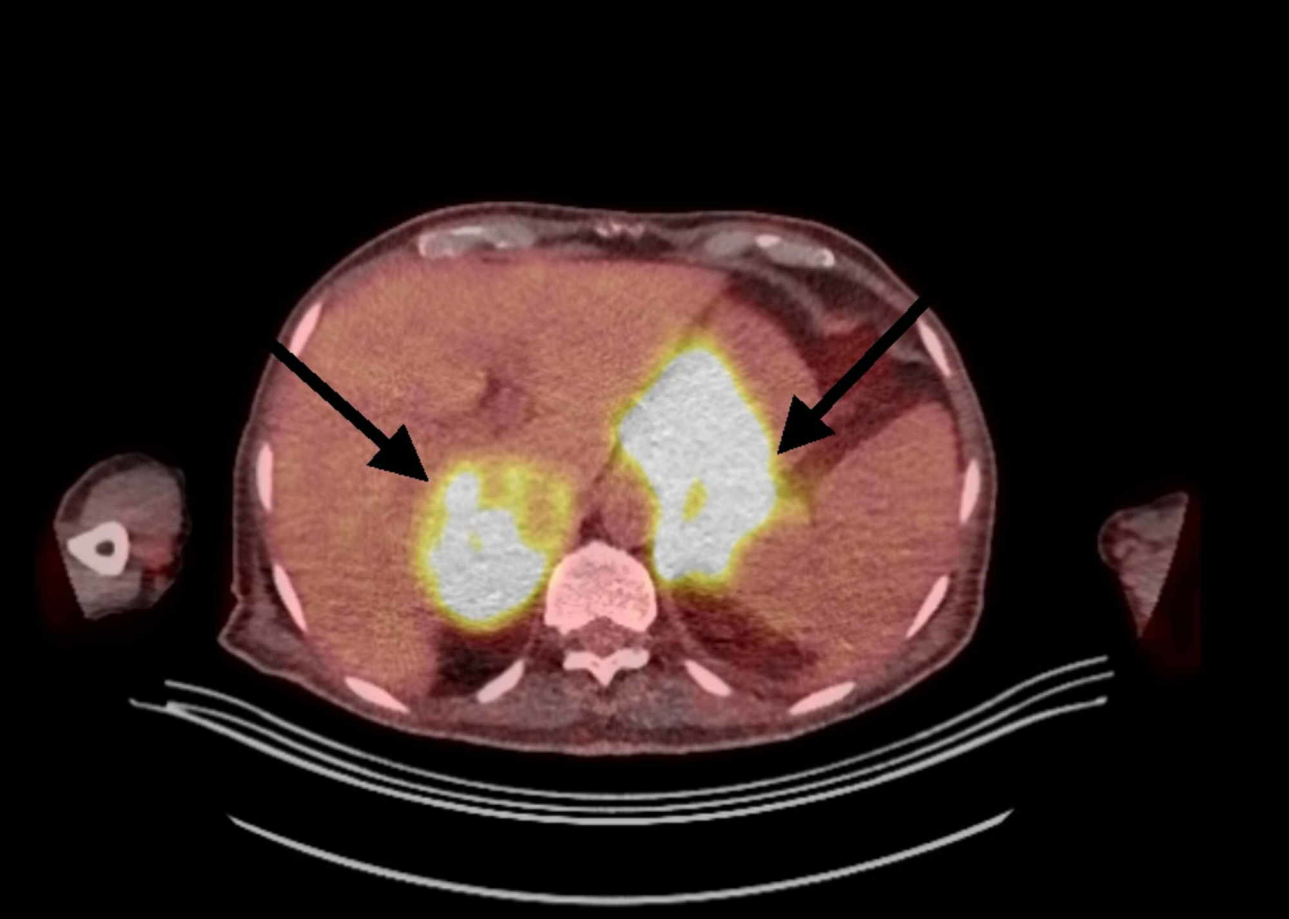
Positron emission tomography/computed tomography (PET/CT) transverse view Bilateral hypermetabolic adrenal glands; on the left side it is extending from the retroperitoneum and mesentery.
